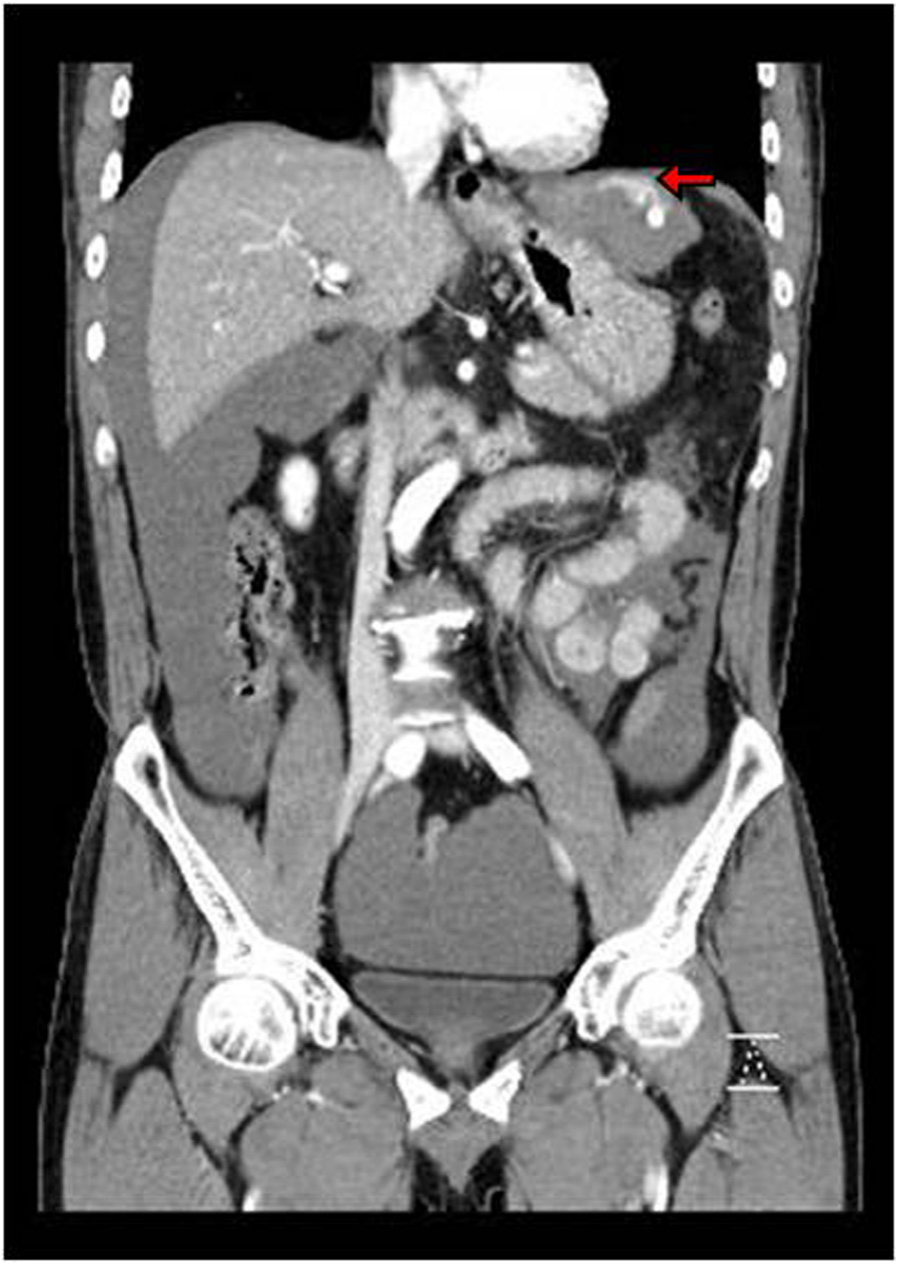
Abdominal CT showes diaphragm invasion of the mass like lesion (arrow).
